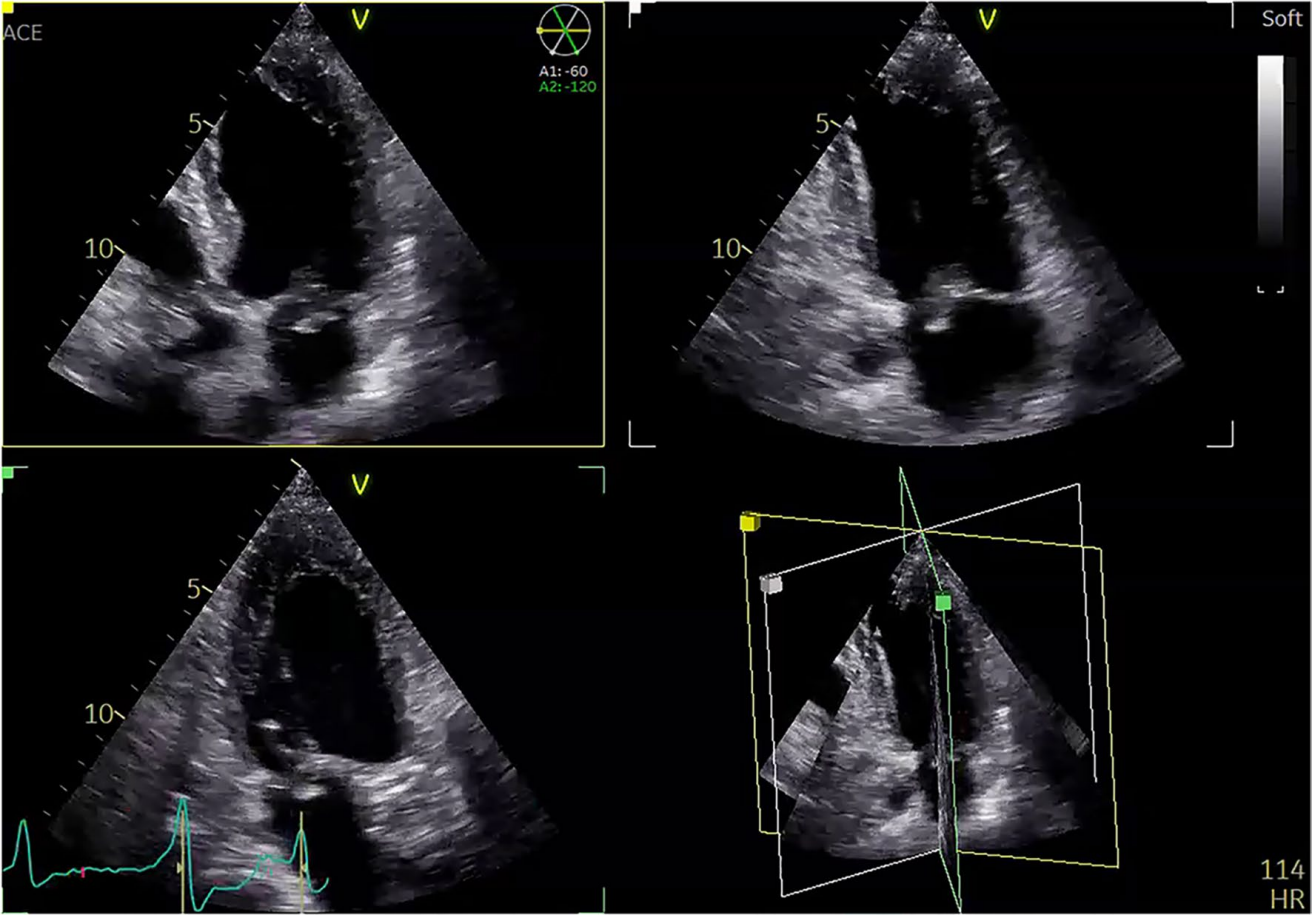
Transthoracal echocardiographic imaging of a mitral valve endocarditis
